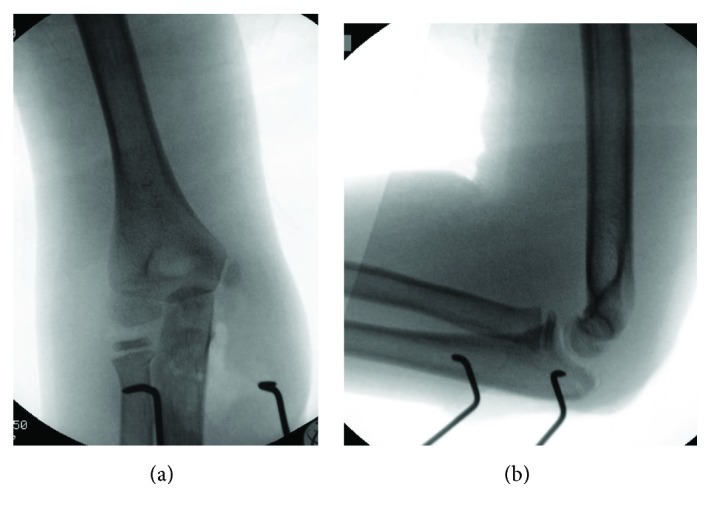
AP (a) and lateral (b) radiographs of the right elbow demonstrating anatomic reduction of both the ulnohumeral and radiocapitellar joints. The radial head fracture has improved alignment.
